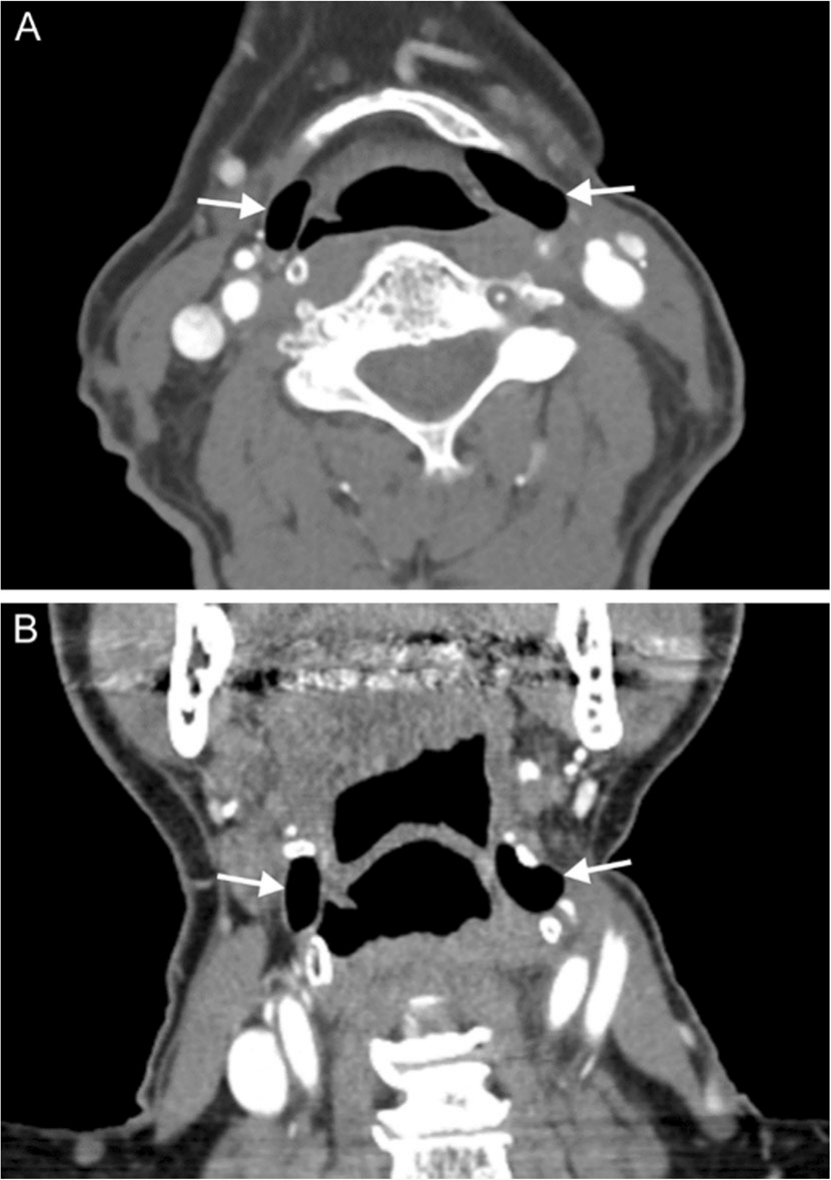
Laryngocele. A 67-year-old man with history of prior tongue base and oropharyngeal squamous cell carcinoma status post radiation completed 8 years prior presents with dysphagia and hoarse voice. Axial (a) and coronal (b) contrast-enhanced CT images demonstrate bilateral supraglottic laryngoceles (arrows) with mild extralaryngeal extension on the left
